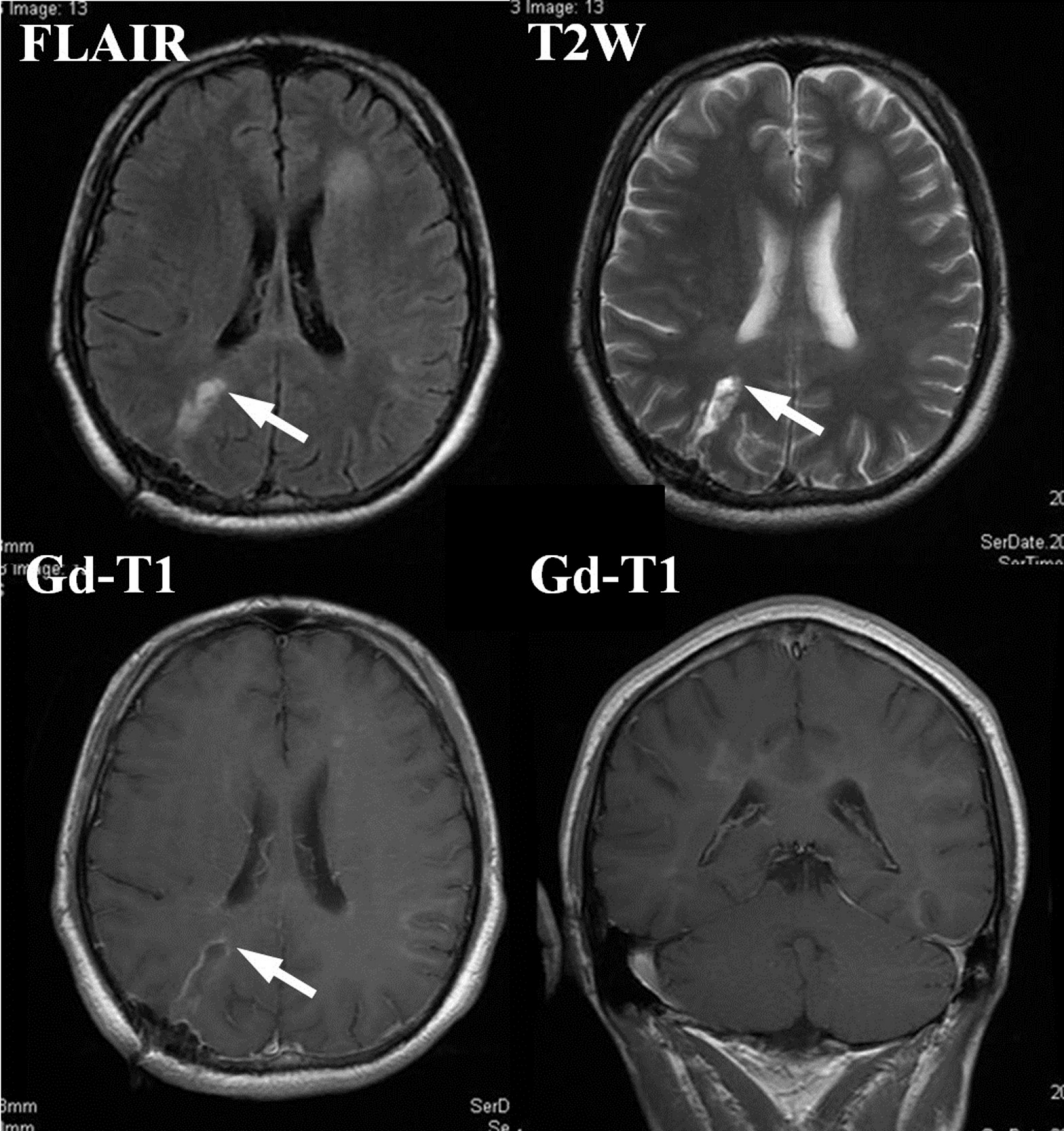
MRI after one month of steroid therapy. High-intensity areas in (FLAIR) and T2W imaging considerably shrink and Gd-enhanced lesions completely disappear after one month of steroid treatment. Arrows show the point of biopsy.
